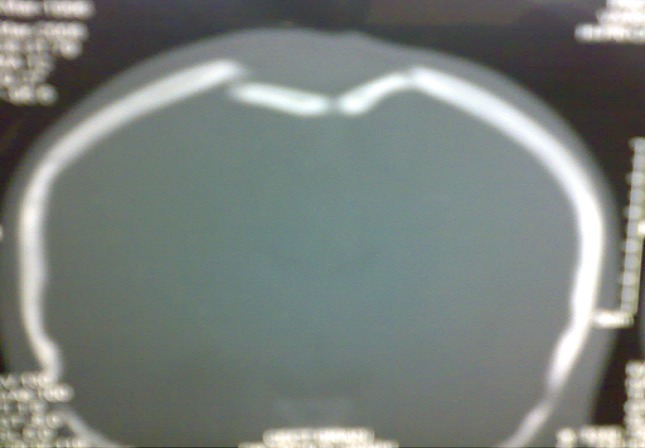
CT scan of the head showing bilateral depressed factures in high parietal area
